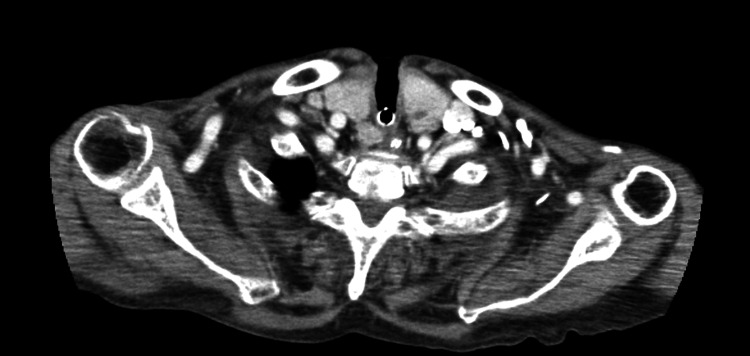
Postoperative contrast-enhanced CT demonstrating disappearance of the pseudoaneurysm after surgical ligation. Axial arterial-phase contrast-enhanced CT obtained after surgical ligation of the right superior thyroid artery shows complete resolution of the previously identified pseudoaneurysm adjacent to the tracheostomy stoma.
